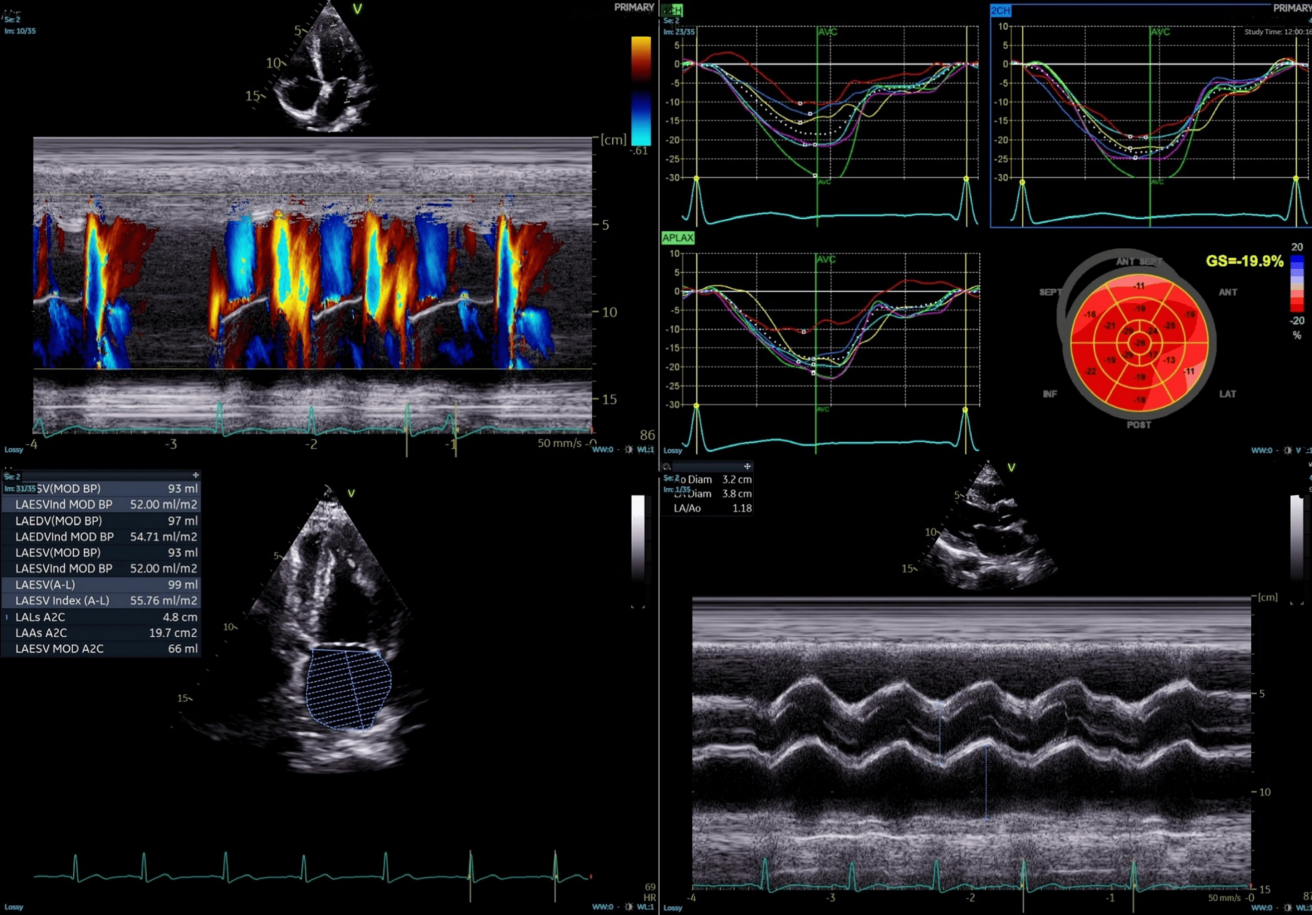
Transthoracic echocardiogram (TTE) TTE after laparotomy and recurrent ventricular arrhythmias shows severe left ventricular dysfunction with an ejection fraction of 25%, likely due to repeated shocks and myocardial stress, consistent with acute systolic dysfunction during an electrical storm.
